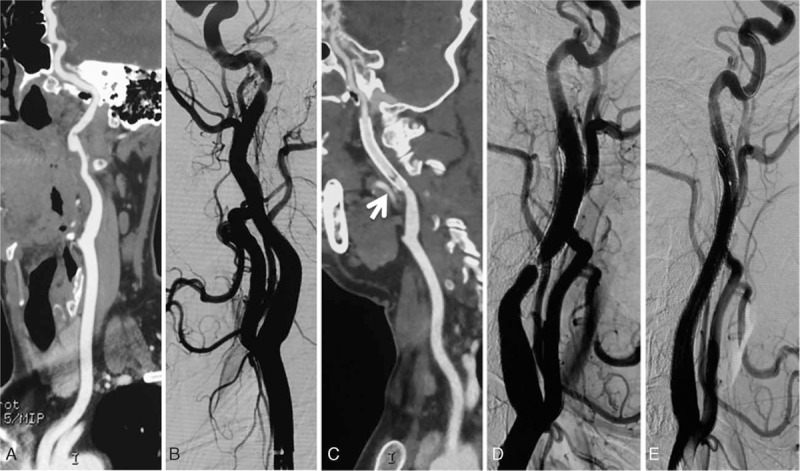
A 45-year-old male suffered from a minor stroke before being diagnosed with a dissecting aneurysm in a high location of the left ICA through CTA examination (A). Endovascular treatment was carried out with deployment of a 6 × 50 mm covered stent (Viabahn, W.L. Gore)(B). Follow-up CTA imaging at 12 months indicated that the level of in-stent restenosis was >70% (marked by white arrows in C). After angiographic assessment (D), an additional stent was placed in the segment of the restenotic lesion after balloon angioplasty with a cerebral protection device in place (E). CTA = computed tomographic angiography, ICA = internal carotid artery.
